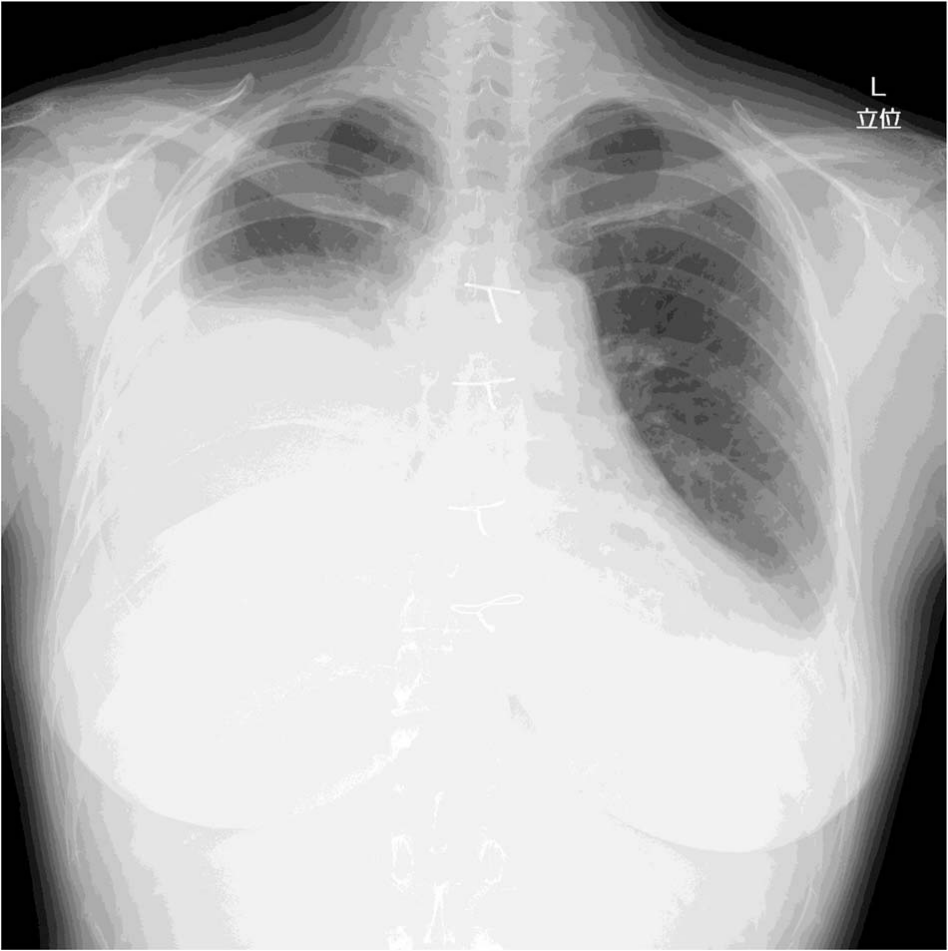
Chest X-ray on post-operative day 17 revealing massive pleural effusion.
